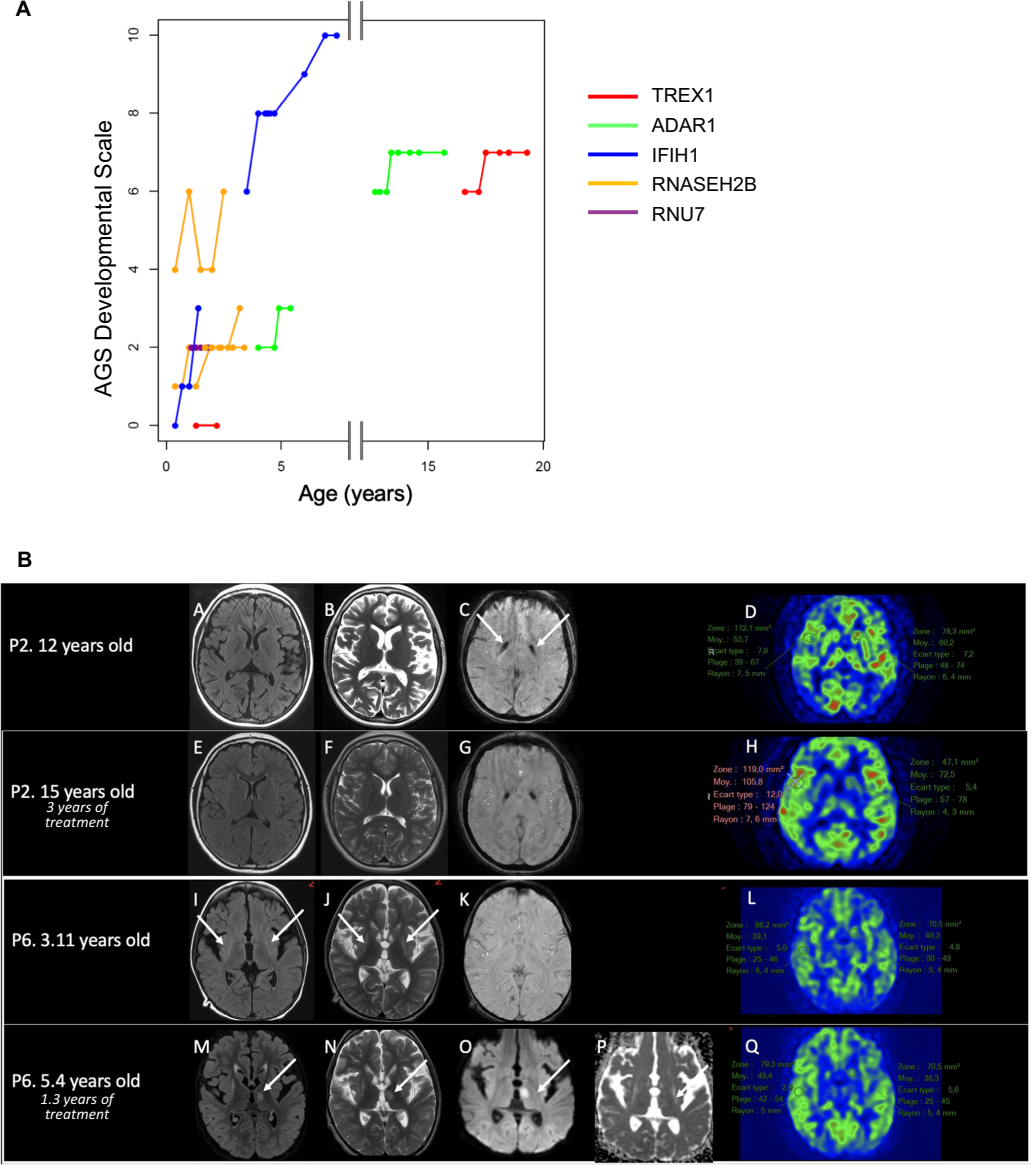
Clinical and radiological response to treatment. A Evolution of the AGS developmental scale [14] in patients under JAK inhibition according to age and mutant genotype. Overall, a slight (mean, 1.2 points) but significant (*p* = 0.036, Kruskal-Wallis test) improvement on AGS severity scale was observed. B Brain magnetic resonance imaging (MRI) in 2 affected patients before and under treatment. The first patient (P2), 12 years old at treatment initiation, demonstrated global supra-tentorial atrophy on axial FLAIR (subpanel A) and T2 (subpanel B)–weighted images associated with calcifications of the globi pallidi on T2 star–weighted images (subpanel C, arrows). After 3 years of treatment, at age 15 years, atrophy had decreased (axial FLAIR (subpanel E), axial T2–weighted images (subpanel F)). On arterial spin labeling sequence (ASL) (subpanels D, H), cortical cerebral blood flow (CBF) increased between the ages of 12 and 15 years (CBF was measured at 53 ml/min/100 g per tissue in the temporal cortex at 12 years (subpanel D), increasing to 105 ml/min/100 g per tissue at 15 years old (subpanel H)). P6 demonstrated bilateral striatal anomalies (arrows) on axial FLAIR (subpanel I) and on axial T2–weighted images (subpanel K) at age 3.11 years. After 1.6 years of treatment, the appearance on the axial FLAIR–weighted (subpanel M) and axial T2–weighted (subpanel N) imaging showed nodular hyperintensity in the left thalamus (arrow). The diffusion-weighted images (subpanel O) showed an increased diffusion coefficient (subpanel P). On ASL (subpanels L, Q), CBF in the basal ganglia and cortex (about 40 ml/min/100 g tissue) (subpanels L and Q respectively) remained unchanged between the 2 periods
